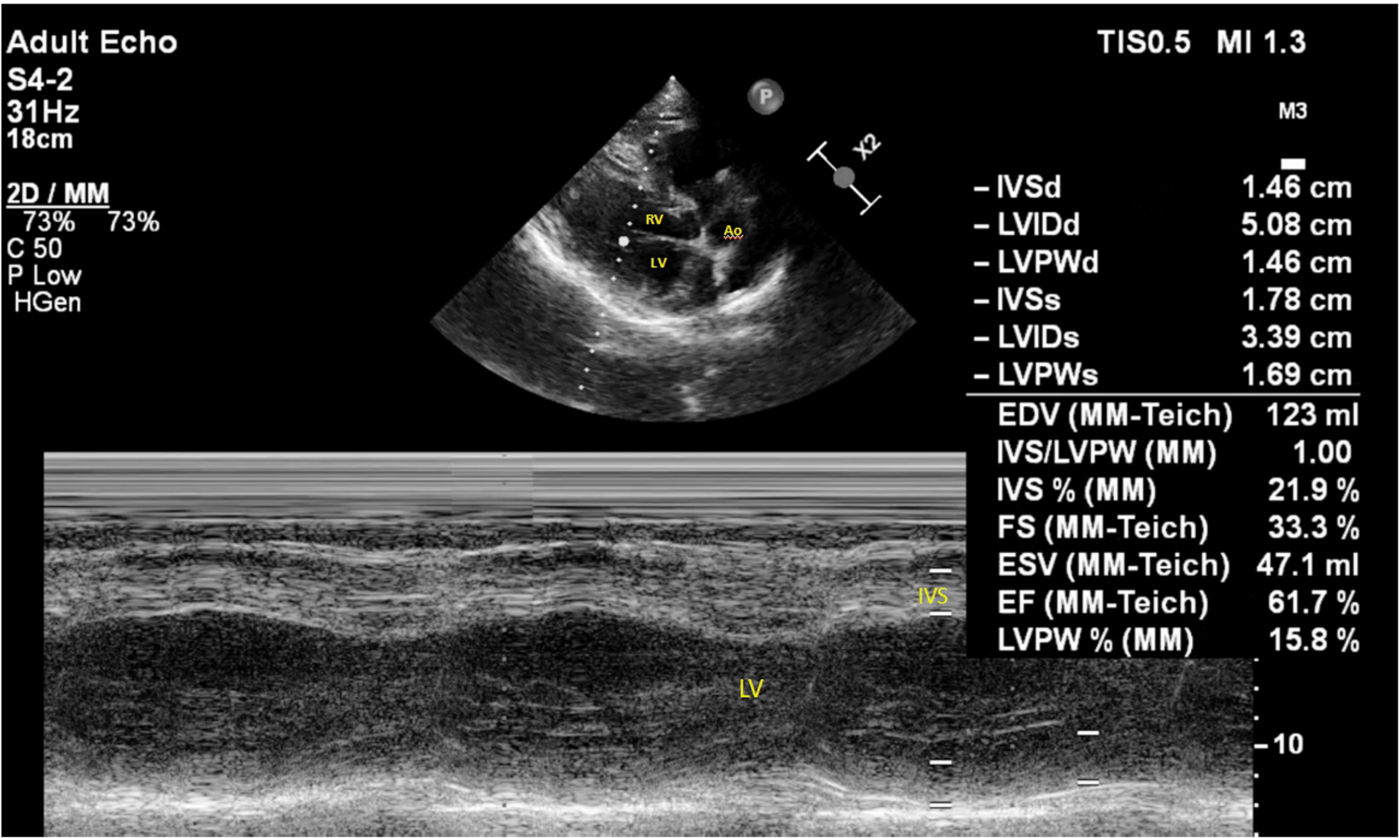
2D echocardiogram demonstrating left ventricular hypertrophy LV - left ventricle; RV - right ventricle; Ao - aorta; IVS - interventricular septum
